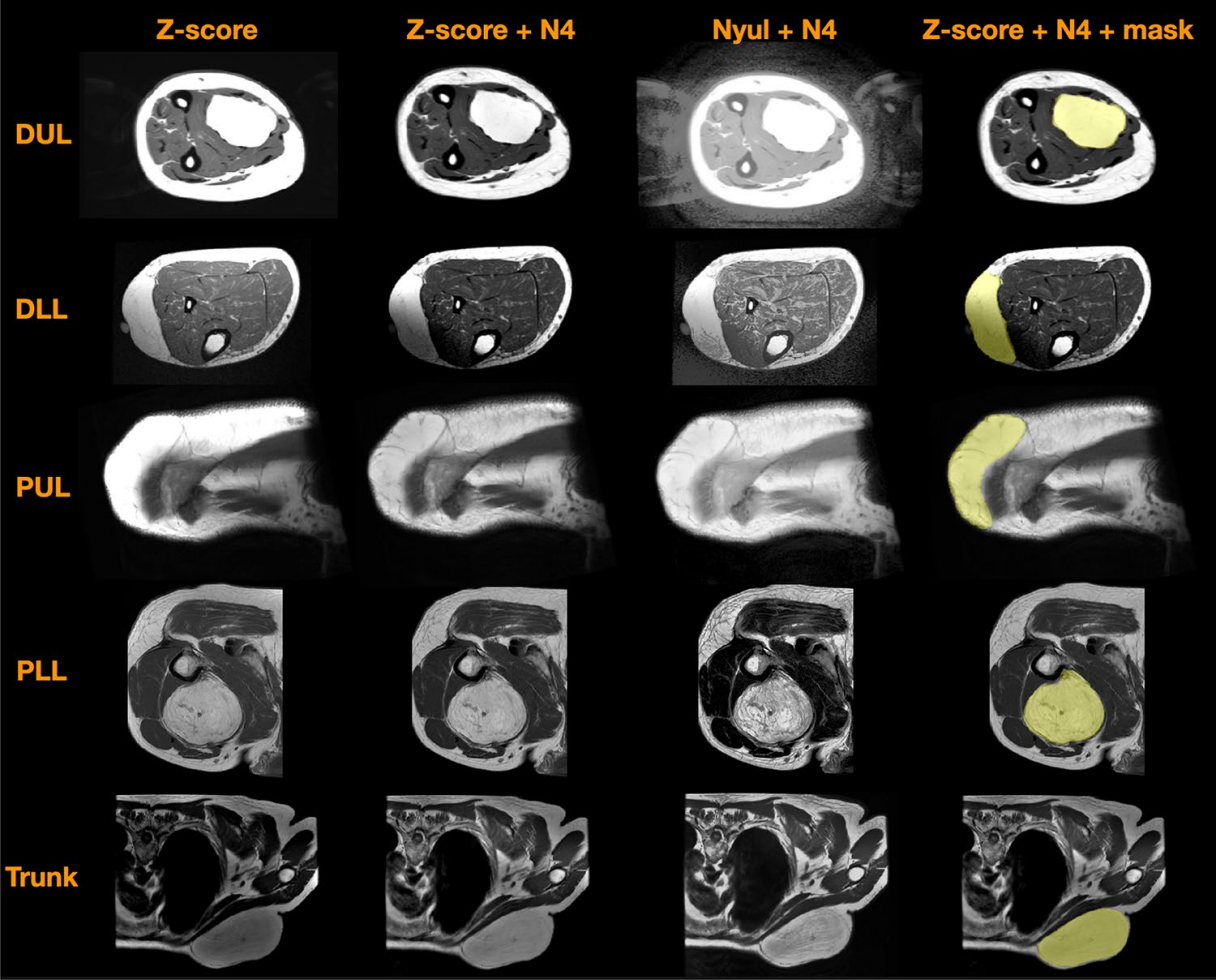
T1-weighted MR images per tumor location (left to right) normalized by Z-score before and after bias correction (N4), normalized by Nyul standardization after N4 correction and the manual delineations (yellow shade) overlaid on MR images. The images are displayed on the same scale. The images (top to bottom) are from distal upper limb (DUL), distal lower limb trunk (DLL), proximal upper limb (PUL), proximal lower limb (PLL), and trunk (T)
